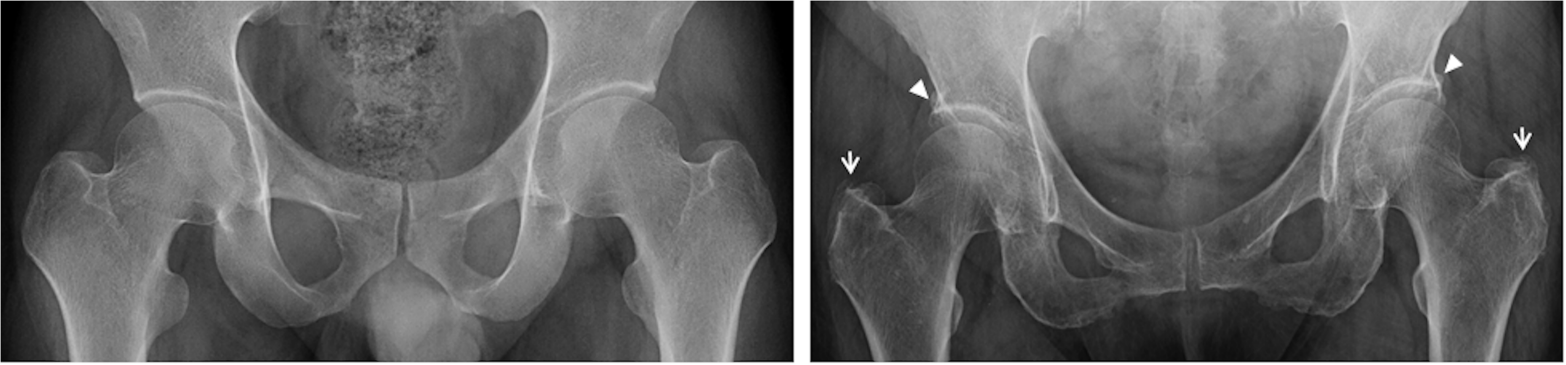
Sample normal and OA hip X-ray images. The images have a pixel resolution of 0.187 mm x 0.187 mm, and were zoomed in on the osteophytes to highlight the differences between normal and OA patients. Left: Normal hip joint X-ray image. Right: OA image. The triangles indicate osteophytes of the acetabular rim bone. The arrows indicate the osteophytes of the greater trochanters of femurs.
